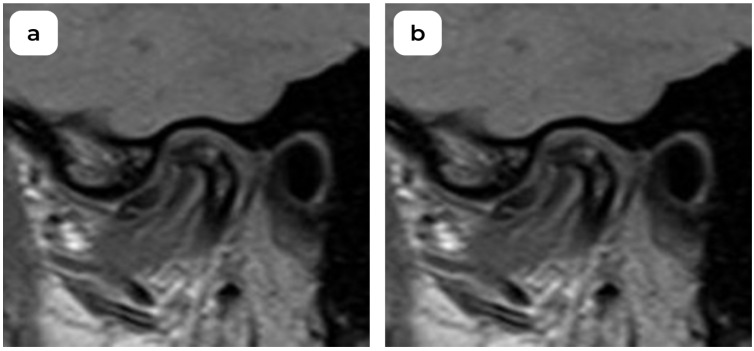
MRI oblique sagittal view of left TMJ: (a) anterior disc displacement in a closed-mouth acquisition, (b) disc displacement in an open-mouth acquisition (DDWoR).
